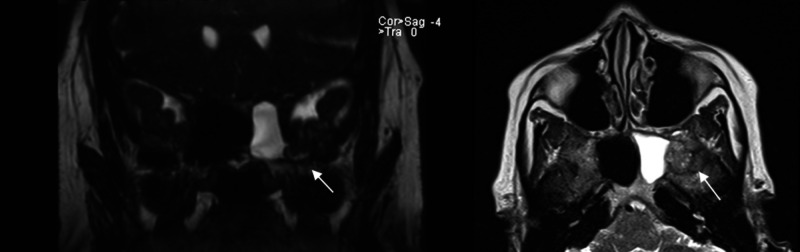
MRI of the brain demonstrating a small transsphenoidal temporal lobe encephalocele through the left lateral craniopharyngeal canal with associated drainage of CSF into the left side of the sphenoid sinus. CSF, cerebrospinal fluid
